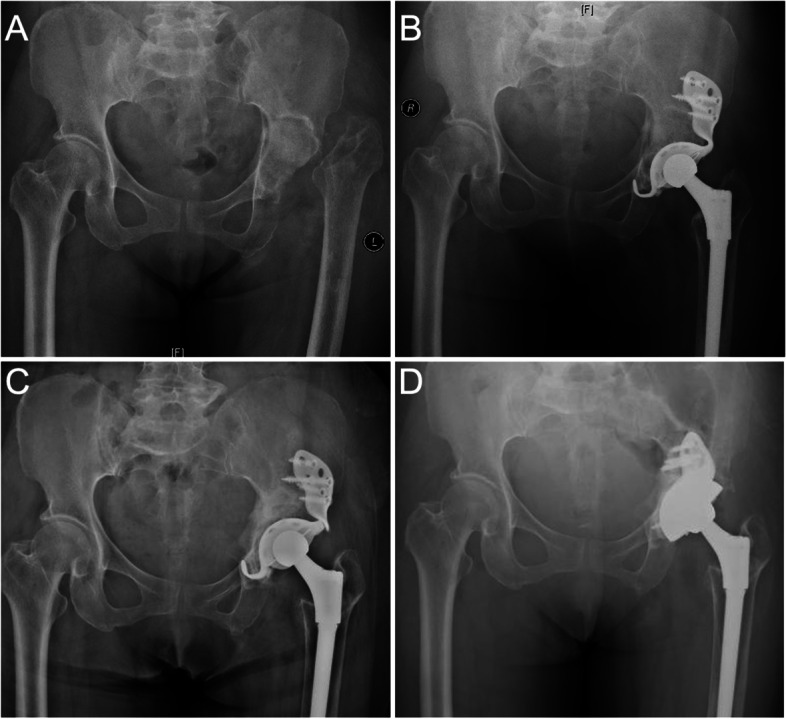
A Pelvis anteroposterior radiograph taken before the first operation. The left femoral neck was fractured. B Radiograph taken right after the primary THA and a commercial cage was used. C 7 years after the first operation, fatigue failure happened in the connection between the wing and cup. D A high friction coefficient revision cup was used in revision
